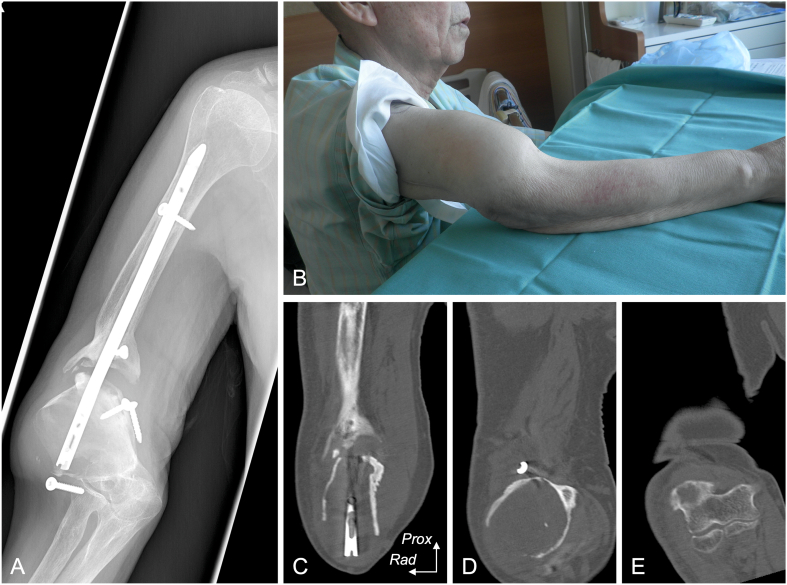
Preoperative findings. A) X-ray revealed nonunion and deformity of the distal fragment. B) Physical findings showed the deformity in the upper arm. C, D) Coronal section of CT showed a bone defect and cavity formation in the distal metaphyseal area. E) The joint surface of the elbow remained intact. *Prox*, *proximal*, *Rad*, *radial*.
